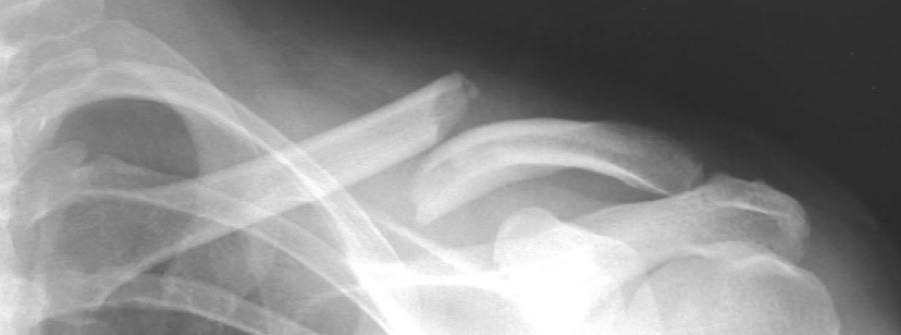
Xray shows a displaced middle third fracture of clavicle.
